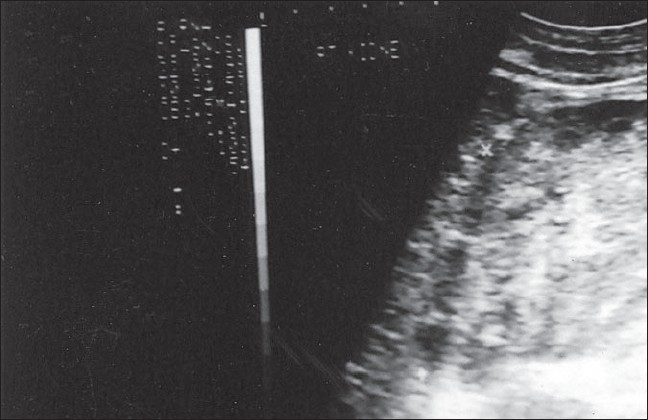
Ultrasound abdomen showing medical renal disease in form of enlarged bright kidneys with poor corticomedullary differentiation
